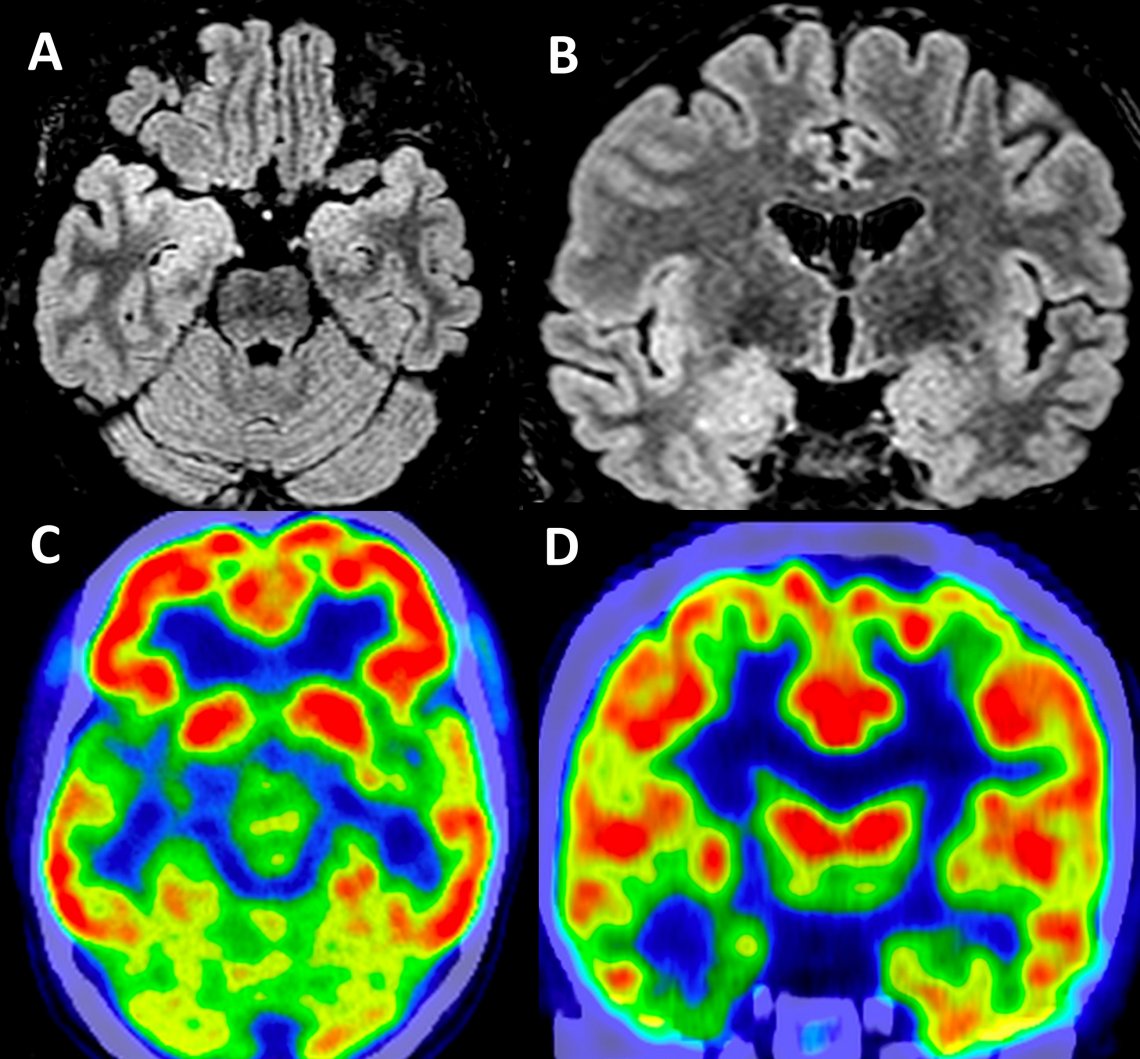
Structural and functional brain imaging in Case 1. Interictal cerebral MRI scan—axial (A) and coronal (B) sections—showing right amygdala and hippocampus FLAIR hyperintensities. Interictal functional imaging using 18FDG-PET—axial (C) and coronal (D) sections—showing extensive right temporal lobe hypometabolism: the temporal pole, mesial and basal areas, and superior temporal and Heschl gyri.
